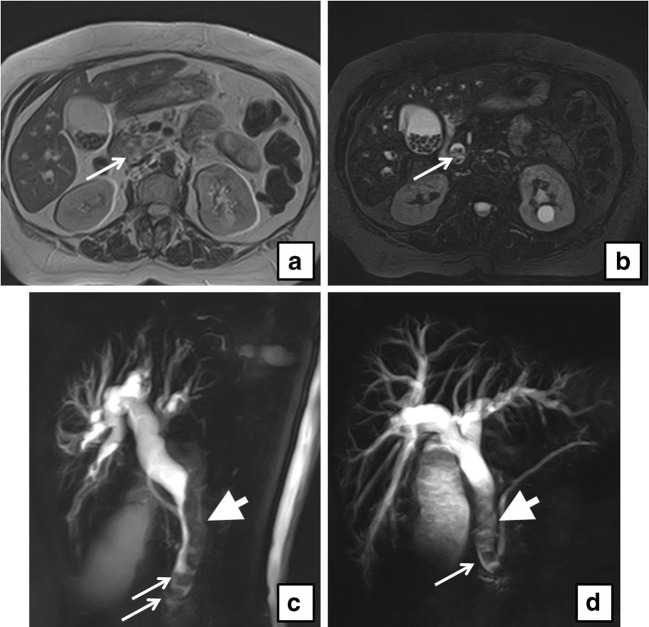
a Axial T2-weighted MRI shows multiple filling defects in the gallbladder and in the dilated CBD (*arrow*) . b Axial fat-saturated T2-weighted MRI of the same patient at the same level of a. c, d Coronal MIP reformat of the same patient shows multiple filling defects in the gallbladder and in the dilated CBD (*arrowhead*), and two stones are seen in the distal CBD (*arrows*)
